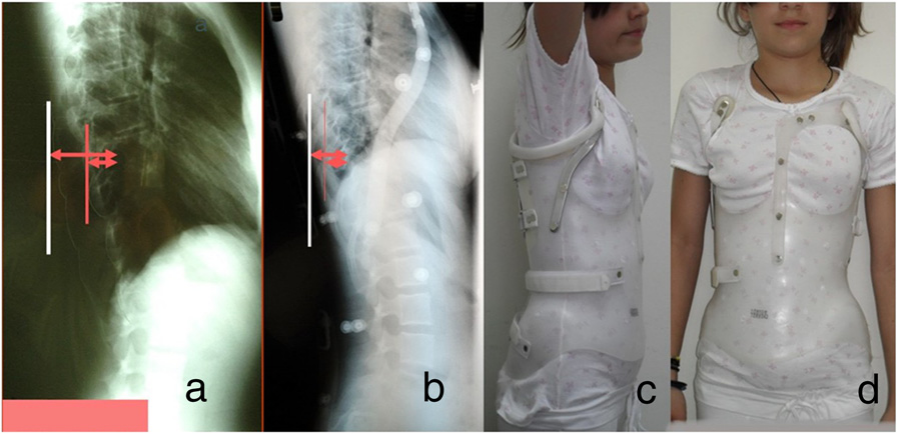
Assessment of the pre-brace treatment RI and the early post-brace. Figure a,b depicts the radiographs and Figure 9
